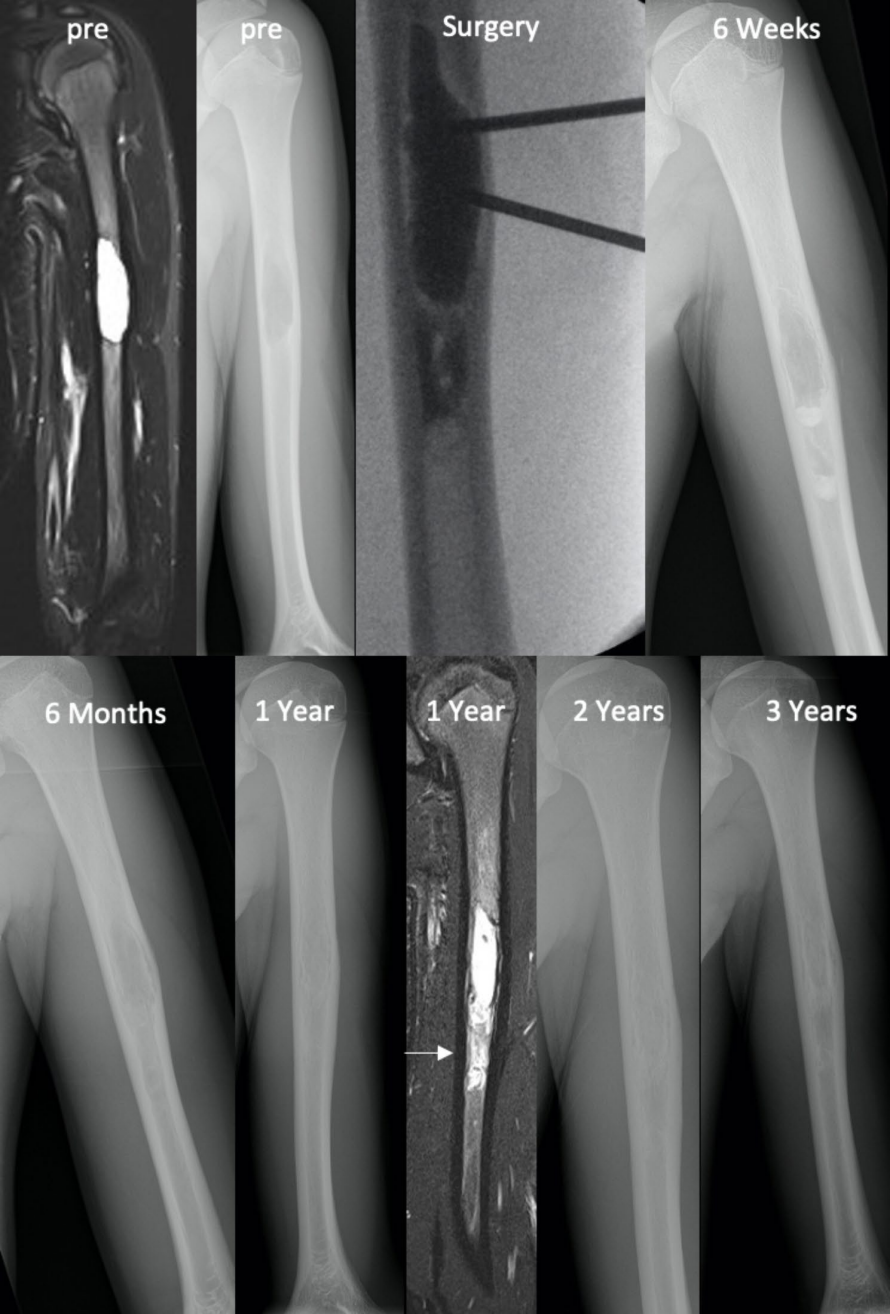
A 15-year-old male patient with a recurring unicameral bone cyst after two open surgeries with curettage and filling with allograft bone chips six years and two years before index surgery. During index surgery, the cyst was percutaneously filled using a two-needle technique. On postoperative X-rays, contrast levels in the distal regions of the cavity could be observed six weeks after surgery, and a double-line phenomenon was visible in the rim area. On MRI one year after surgery, a T2 hyperintense signal with a cylindric filling core could be observed (white arrow). Gradual bony consolidation was found on follow-up X-rays, with full consolidation two years after index surgery
